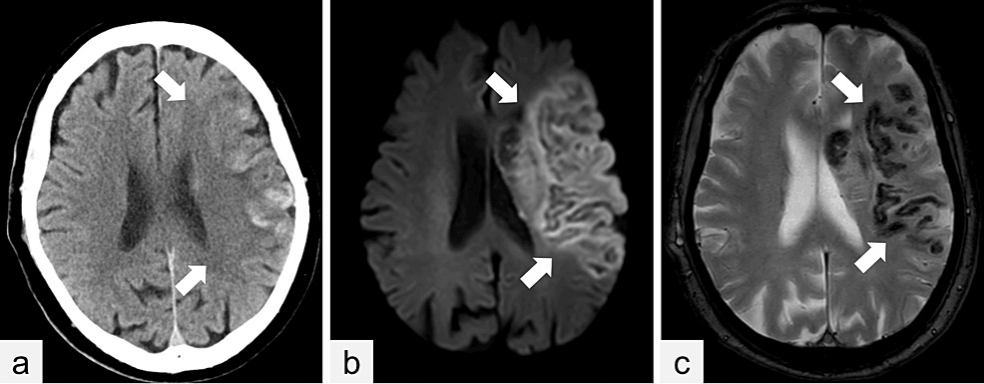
Postoperative computed tomography and magnetic resonance imaging (a) Postoperative computed tomography imaging shows a hypodense region within the left cerebral hemisphere (arrows). (b) Postoperative magnetic resonance imaging shows an extensive middle cerebral artery territory infarction on diffusion-weighted image (arrows) and (c) intracerebral hemorrhage on T2 image (arrows).
